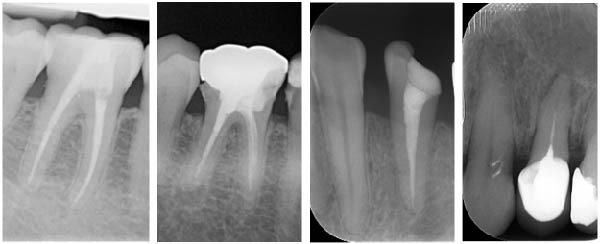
Examples of radiographs (from left to right). GE, good endodontic treatment; GR, good restoration; PE, poor endodontic treatment; and PR, poor restoration.
